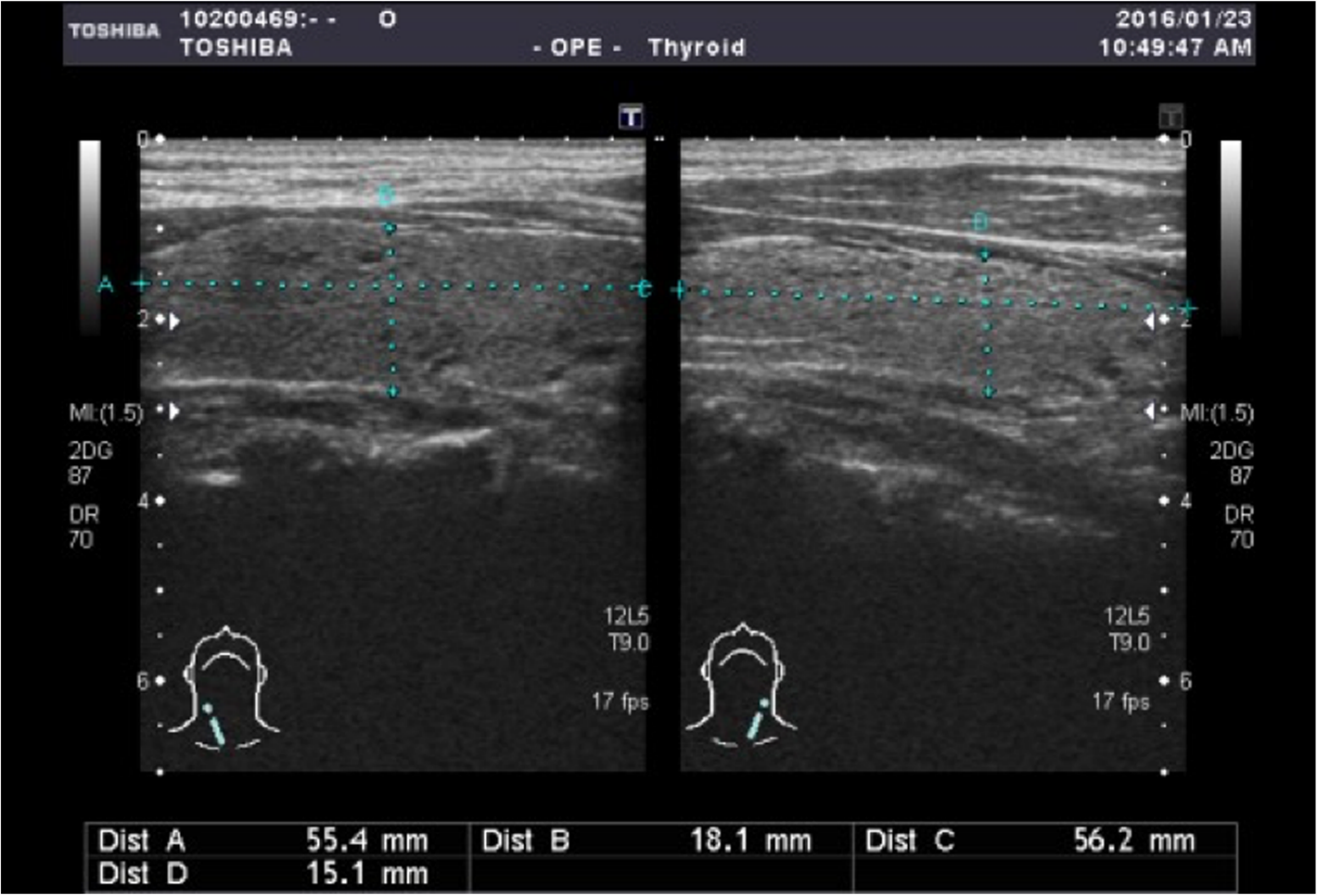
Thyroid sonogram, Right thyroid: 5.62 X 1.51 cm, Left thyroid: 5.54 X 1.81 cm
